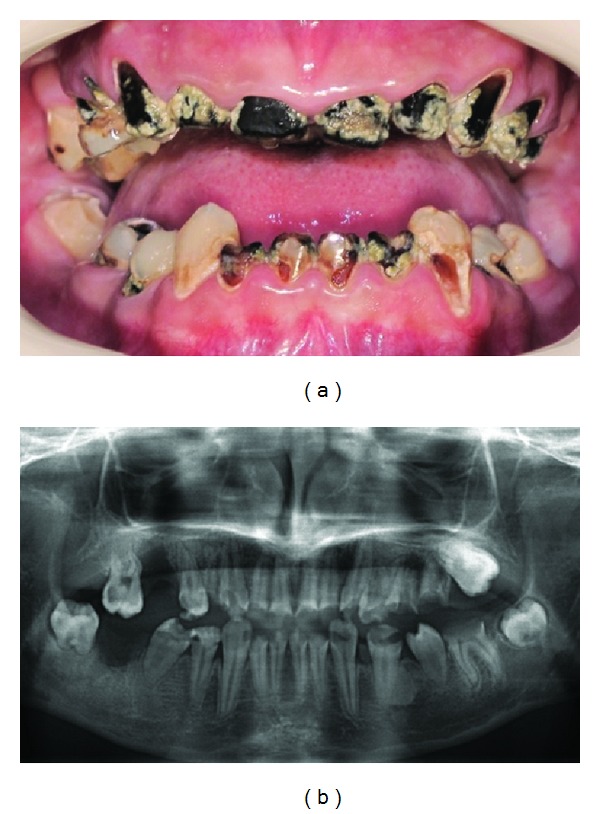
Initial intraoral (a) and radiographic (b) view of the patient.
